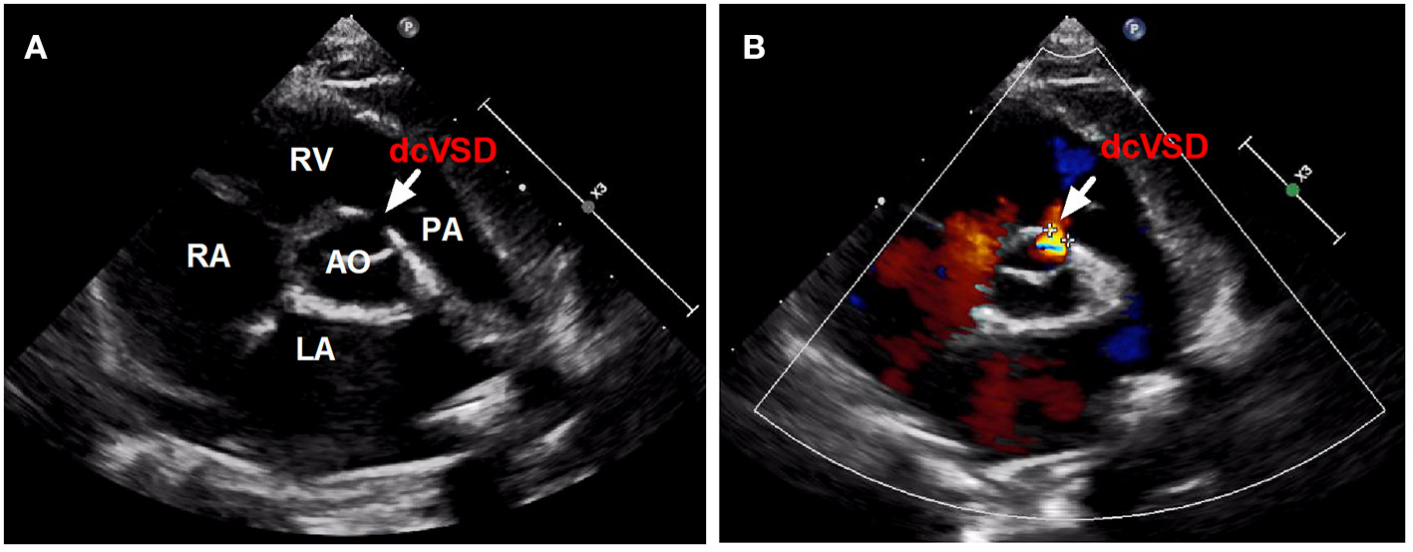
The confirmed diagnosis of dcVSD by TTE. Parasternal short-axis view (A,B) on TTE shows the dcVSD located at the 1-2 o'clock position (arrows). AO, aortic; LA, left atrium; RV, right ventricle; RA, right atrium; PA, pulmonary artery; dcVSD, doubly committed subarterial ventricular septal defect; TTE, transthoracic echocardiography.
